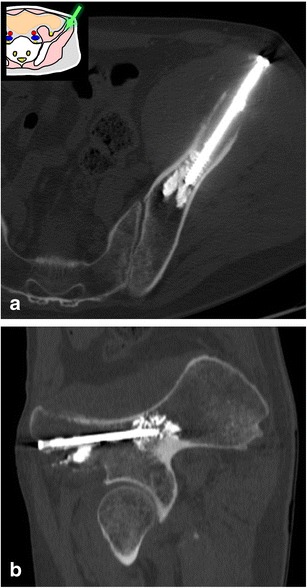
A 75-year-old man treated for a rectal adenocarcinoma presenting with a single painful metastasis in the left iliac bone and early pathological fracture. A cementoplasty combined with percutaneous osteosynthesis was performed along the greater axis of the iliac wing. a Axial CT and b sagittal oblique reconstruction show the screw and the cement in the iliac bone
